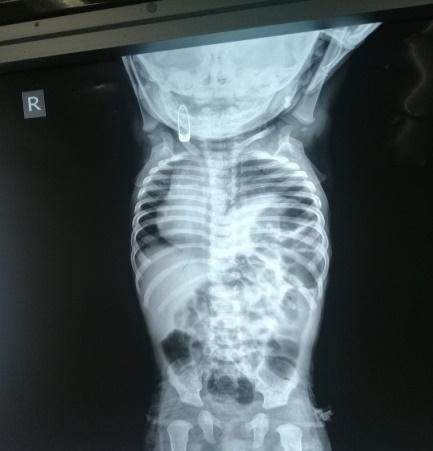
Chest X-ray showing elevated dome of left hemidiaphragm with cardiac silhouette towards the right side.
